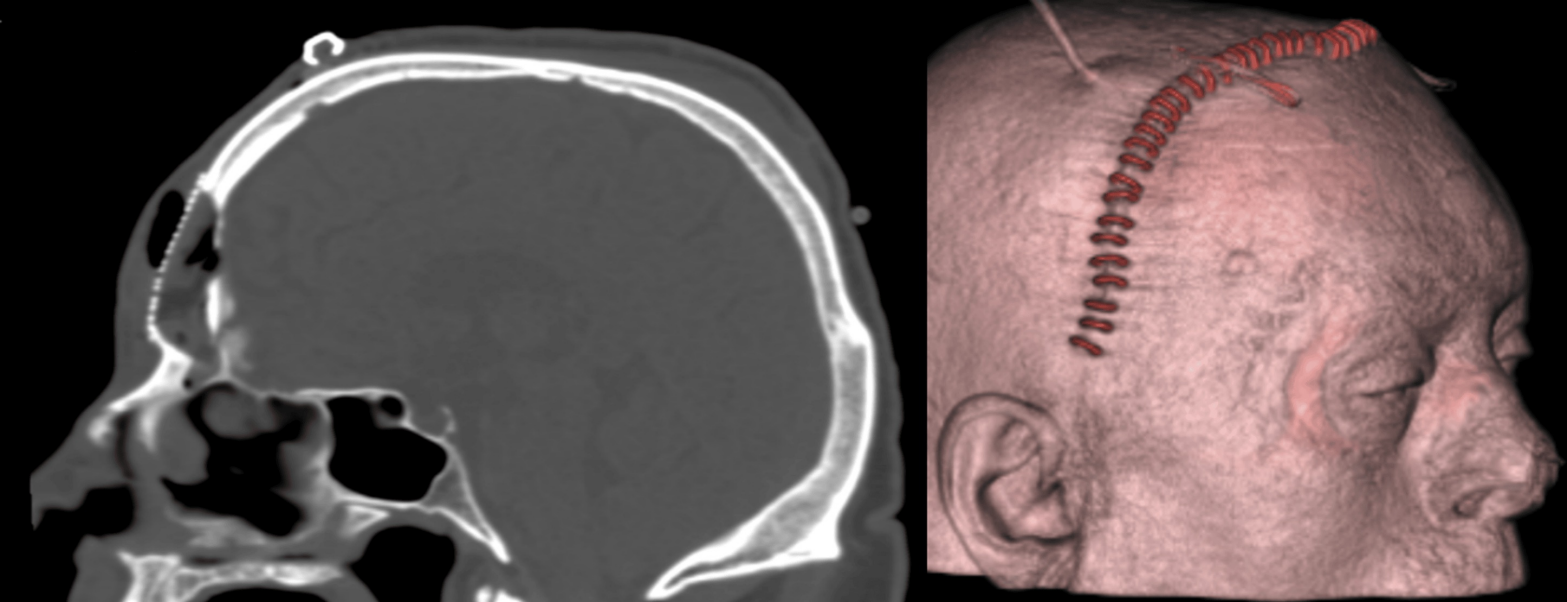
Post-op sagittal CT scan and surface rendering after the removal of the mass and bone reconstruction. CT: computed tomography
